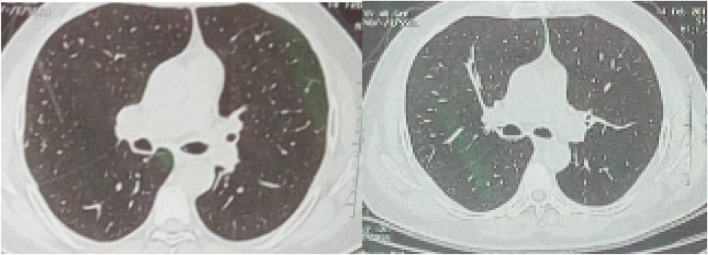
Base line and follow up HRCT of patient in whom BLVR was done with fibrin glue at 1^st^ month post procedure
